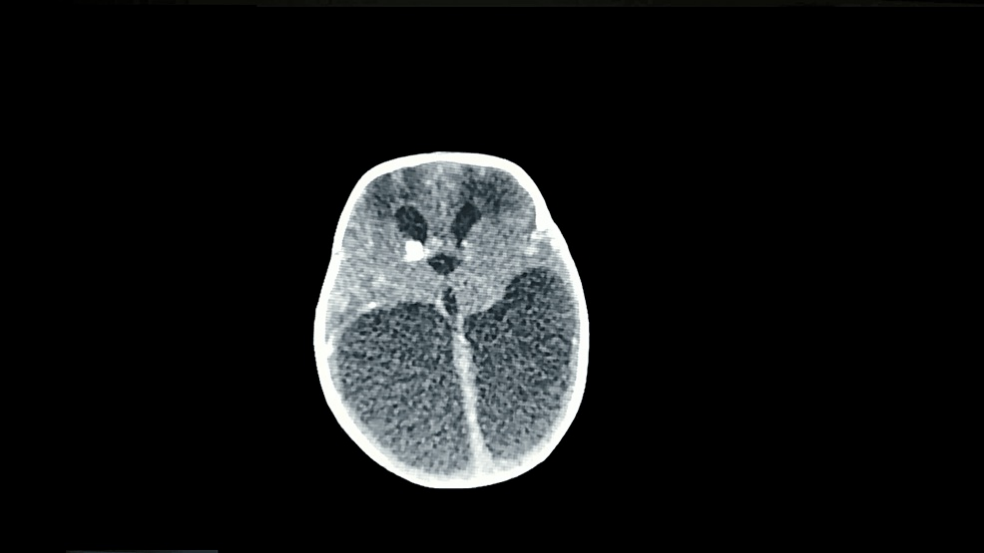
Head CT scan showing colpocephaly with multiple periventricular calcifications
